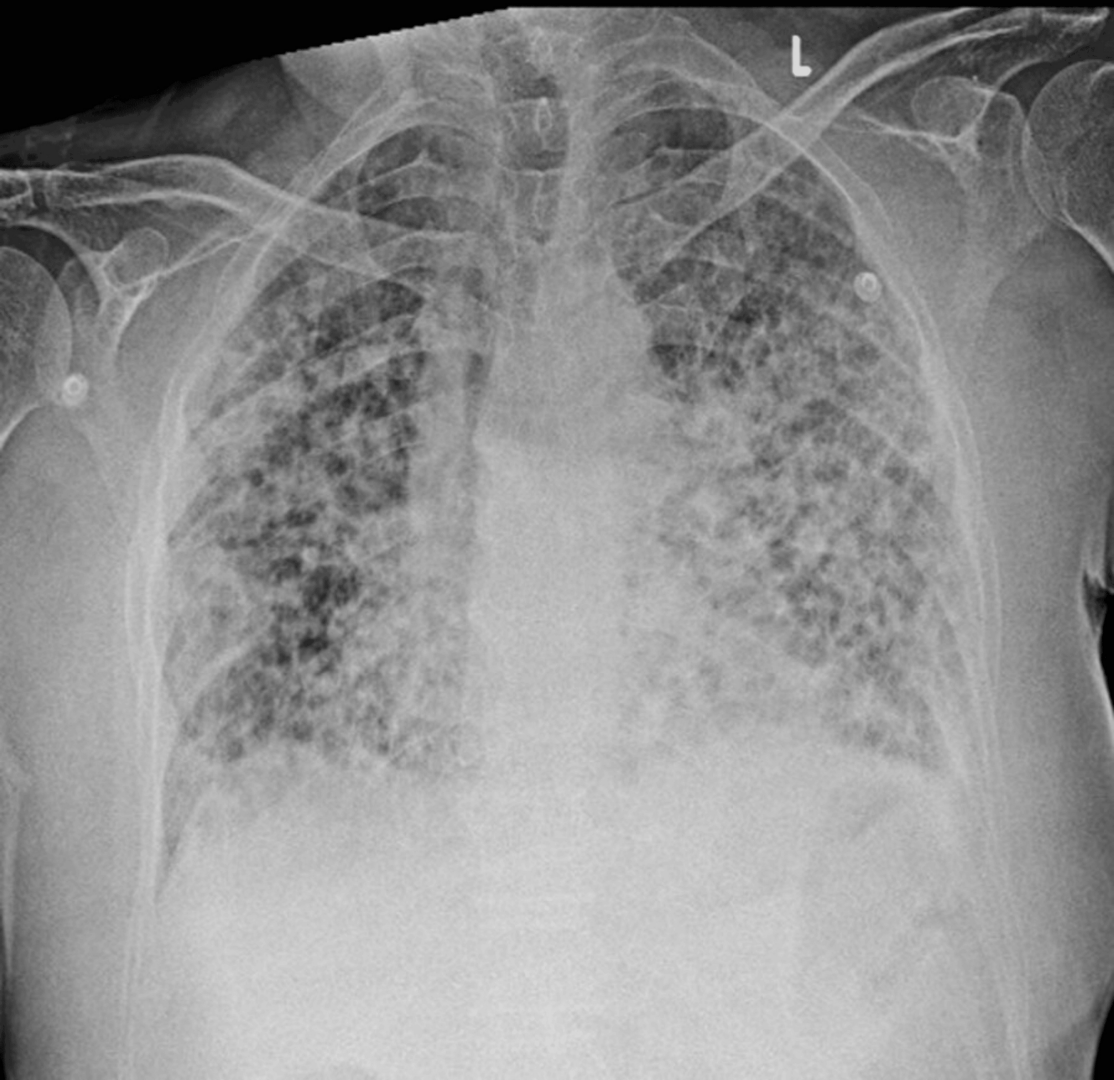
Chest X-ray showing bilateral upper, mid and lower zones reticular opacities
